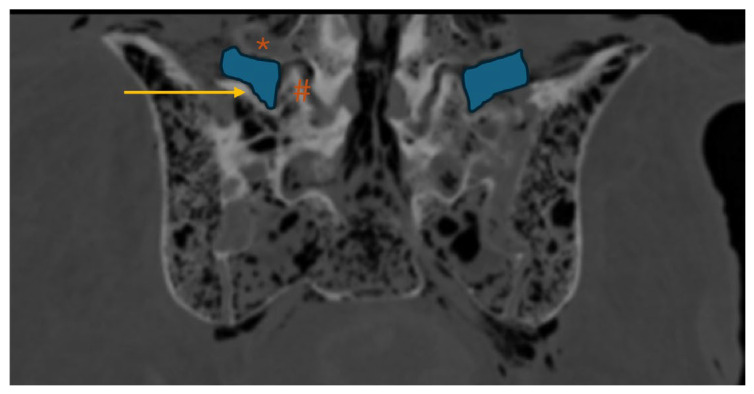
Lumbosacral spine CT—coronal plane; *: L5 transverse process (TP); #: L5–S1 facet joint; arrow: sacral ala; blue area: EF (extraforaminal) area (EF area bounded superiorly by the L5 TP, medially by the L5–S1 facet joint and inferiorly by the sacral ala).
